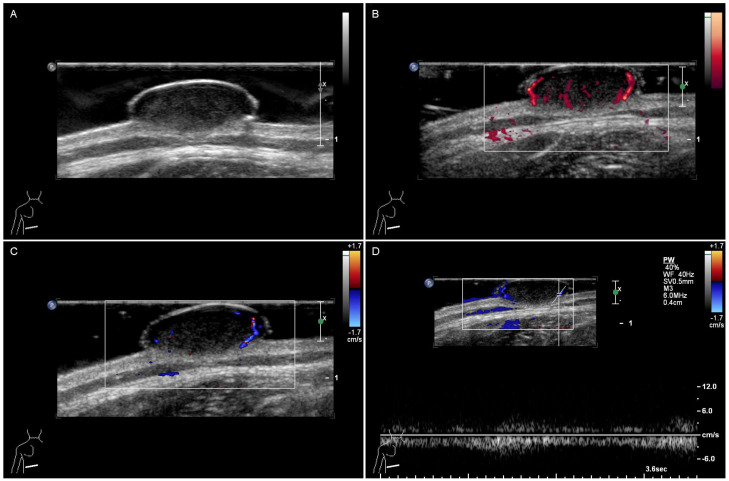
Conventional ultrasonographic features of a typical JXG lesion: (A) B-mode imaging reveals a hypoechoic mass with well-defined borders and a regular shape; (B,C) power Doppler imaging and color Doppler flow imaging detect internal and peripheral vascularity with scattered flow signals; (D) pulsed-wave Doppler analysis confirms a venous flow pattern characterized by continuous, low-velocity waveforms.
